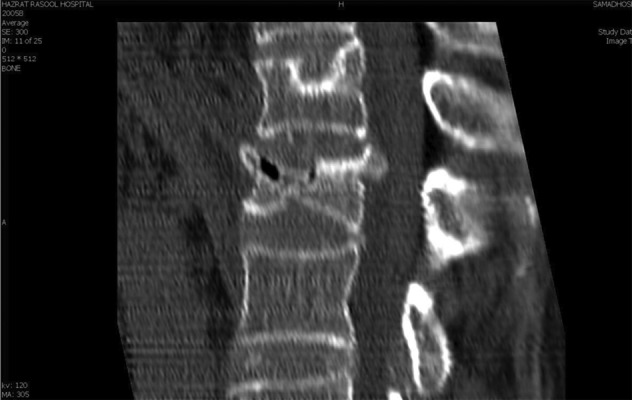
CT Scan of Lumbar Vertebral Column With L1 Vertebral Compression Fracture
